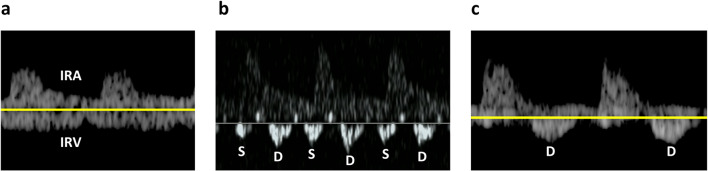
a A normal intrarenal Doppler pattern exhibits arterial pulsations generating a regular positive deflection wave, and IRV generating a continuous, smooth negative deflection wave. As renal venous congestion increases, venous pulsations become visible. b In mildly abnormal waveforms, venous pulsations with clearly visible anterograde S and D waves are present. c Severely abnormal waveforms display a retrograde S wave with only an anterograde D wave; *IRA* Interlobar Renal Artery, *IRV* Interlobar Renal Vein
